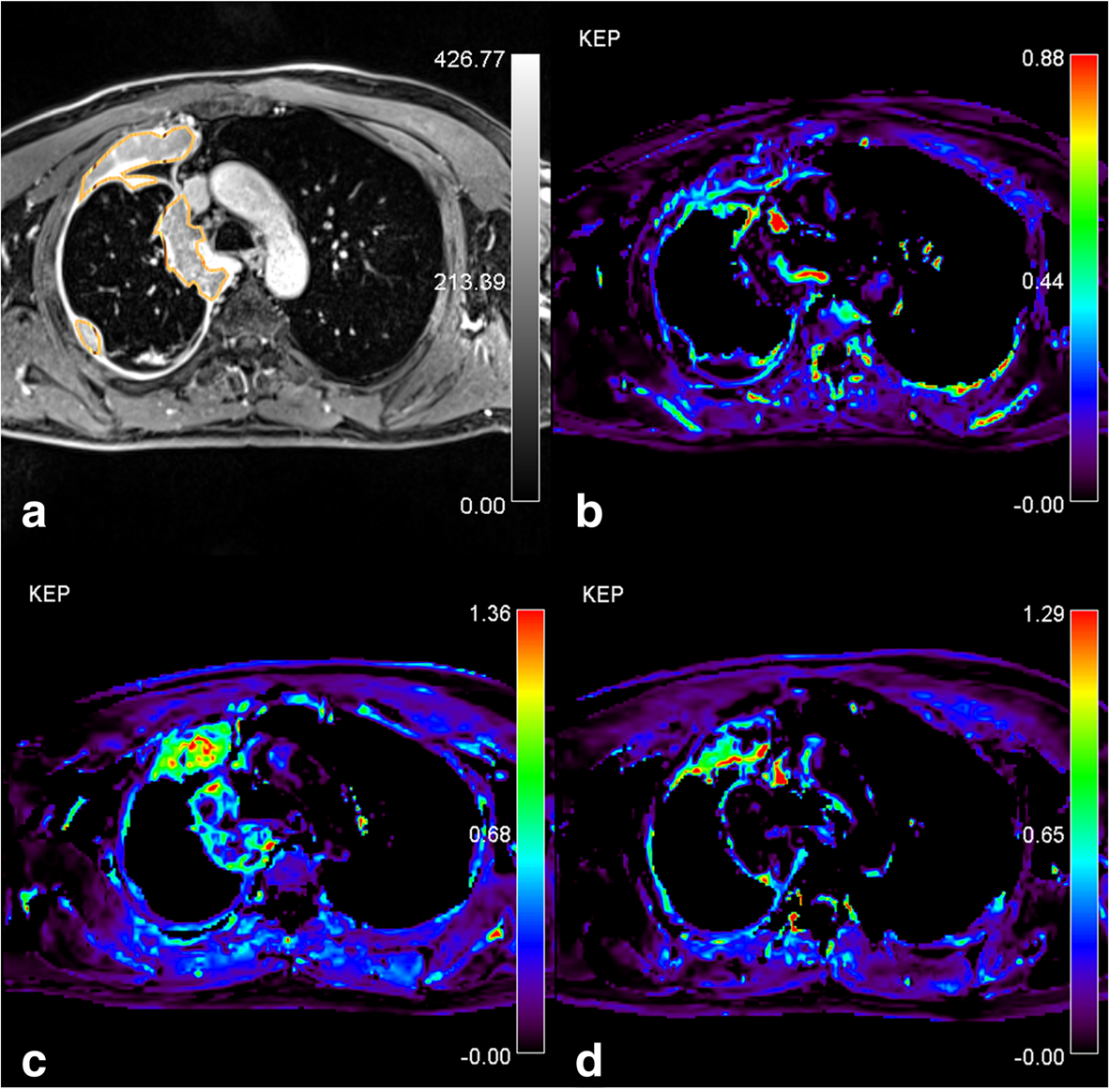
Patient with progressive disease. a) Post-contrast T1-weighted turbo-FLASH. Source image shows the region of interest (ROI) drawn around the tumor, AATH-calculated k_ep_ parametric map (1/min) from b) pre-treatment c) intra-treatment and d) post-treatment study are shown. At intra-treatment study, tumor showed an increase in k_ep_ as well as in size. At the post-treatment study, a decrease in k_ep_ and a slight increase in tumor size is seen. Significant heterogeneity of the tumor can be observed
